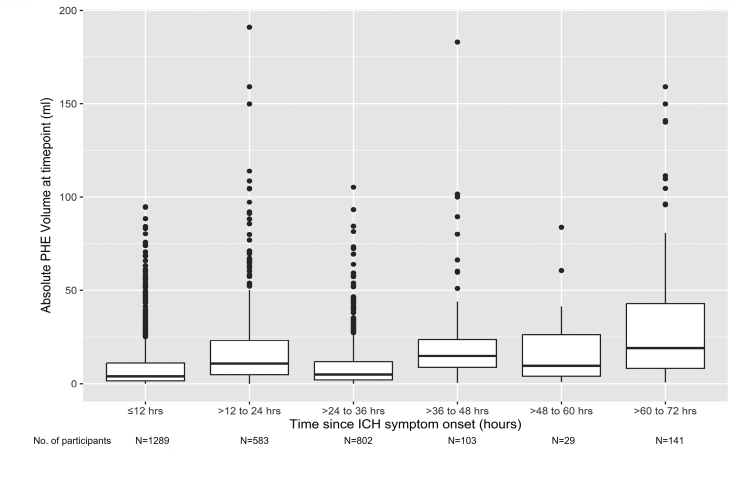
Box and whisker plot of absolute perihematomal edema PHE volumes in milliliters (mL) in 12 hourly intervals over the first 72 hours after intracerebral hemorrhage (ICH) onset. Data at each time point are based on the subset of participants in whom computed tomography was performed at that time point.
